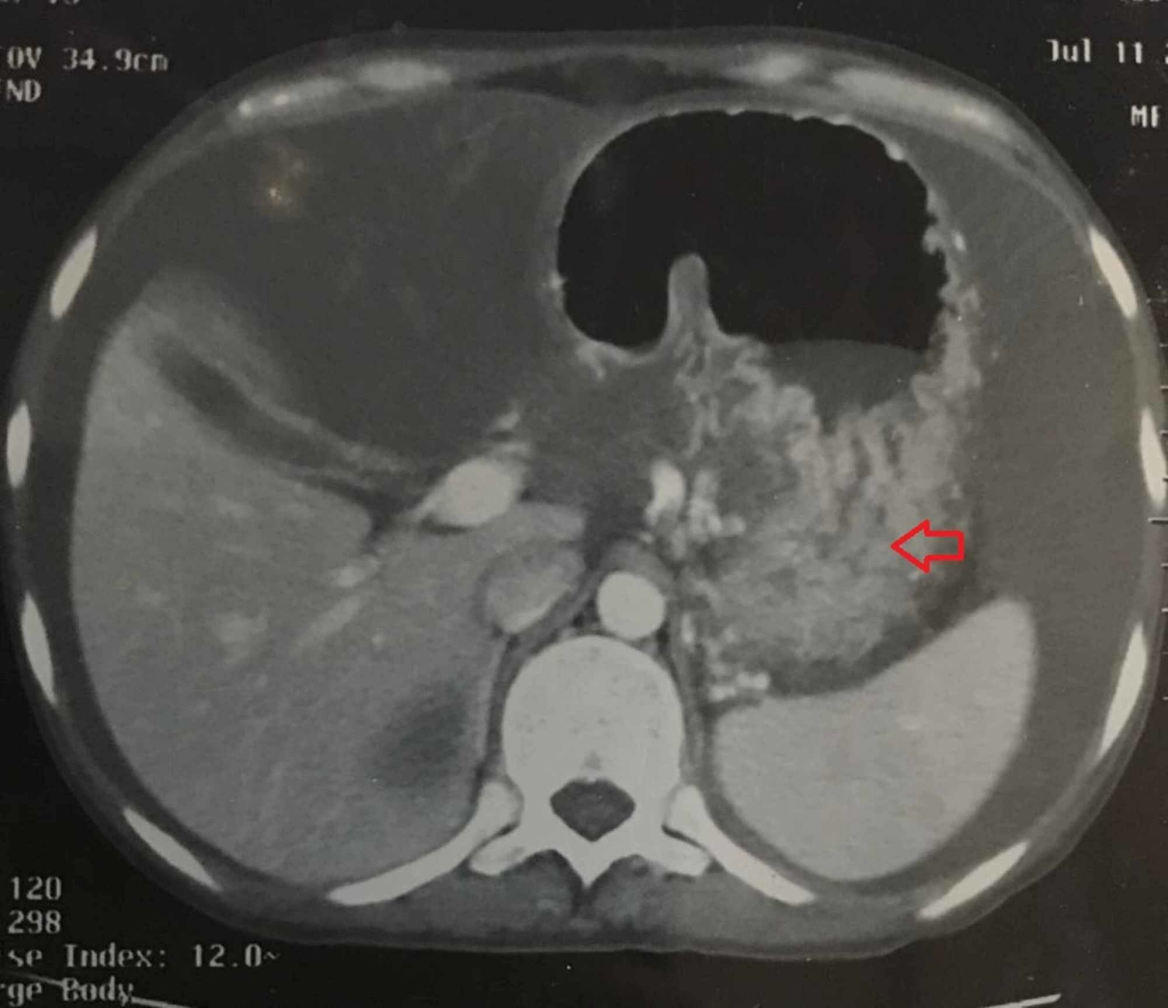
Abdominal CT scan showing cystic collection
